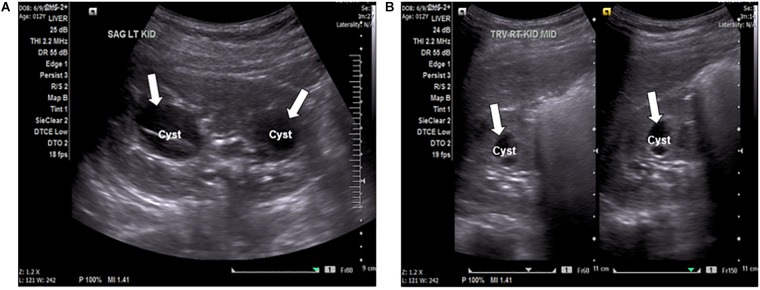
(A) Ultrasound of the left kidney, sagittal view, showing anechoic fluid-filled septated cystic spaces (arrows) insinuating the renal sinuses. The cyst on the left is an image of the largest cyst present in the kidneys, measuring 3.3 mm. (B) Ultrasound of the right kidney, transverse view, showing anechoic fluid-filled multi-septated spaces (arrows).
